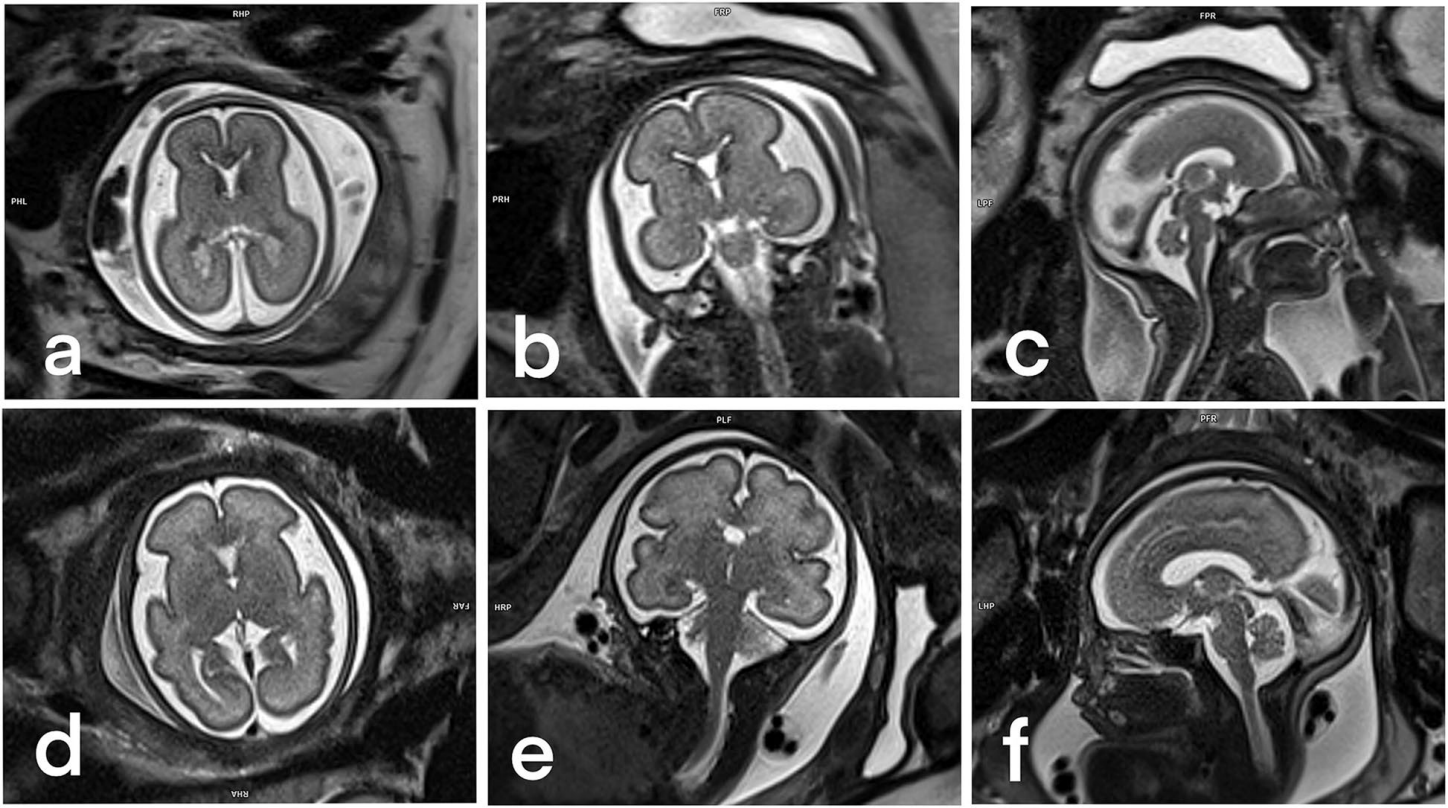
Transversal, coronal, and sagittal views of a T2-weighted single-shot fast spin-echo optimized sequence in fetuses of 22 weeks (a, b, c) and 28 weeks (d, e, f) 28 weeks
